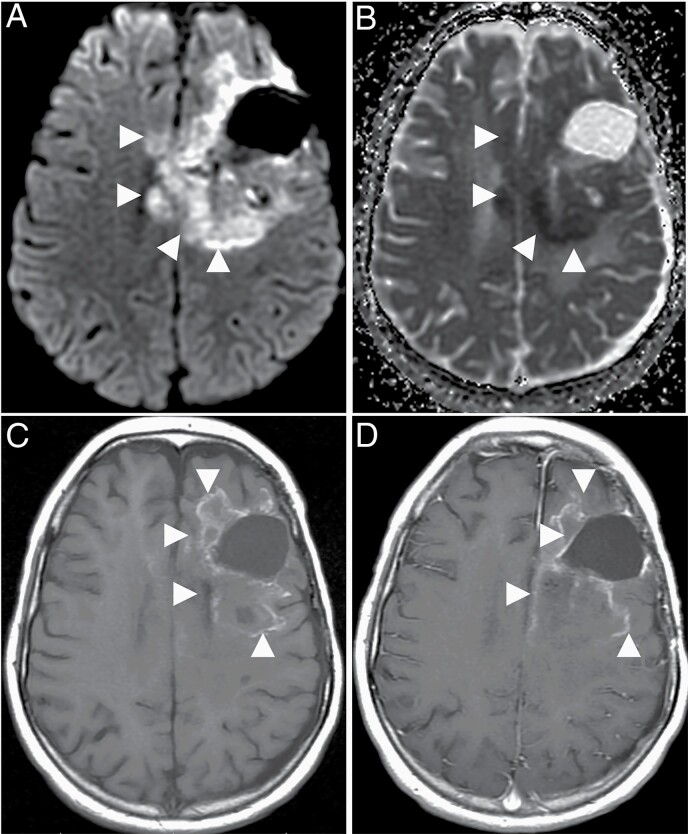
Imaging appearances of a glioblastoma following treatment with bevacizumab. Axial diffusion weighted image (A) through the level of centrum semiovale demonstrates a confluent area of high signal (arrowheads) surrounding the cystic resection cavity associated with low values (arrowheads) on the corresponding ADC map suggestive of diffusion restriction developed after treatment with bevacizumab. The precontrast axial T1-weighted image (C) at the same level demonstrates irregular T1 hyperintensities. Enhancement, if any, is hard to appreciate on the post contrast T1 weighted image (D) at the same level, demonstrating the importance of the delta T1 map (not shown).
